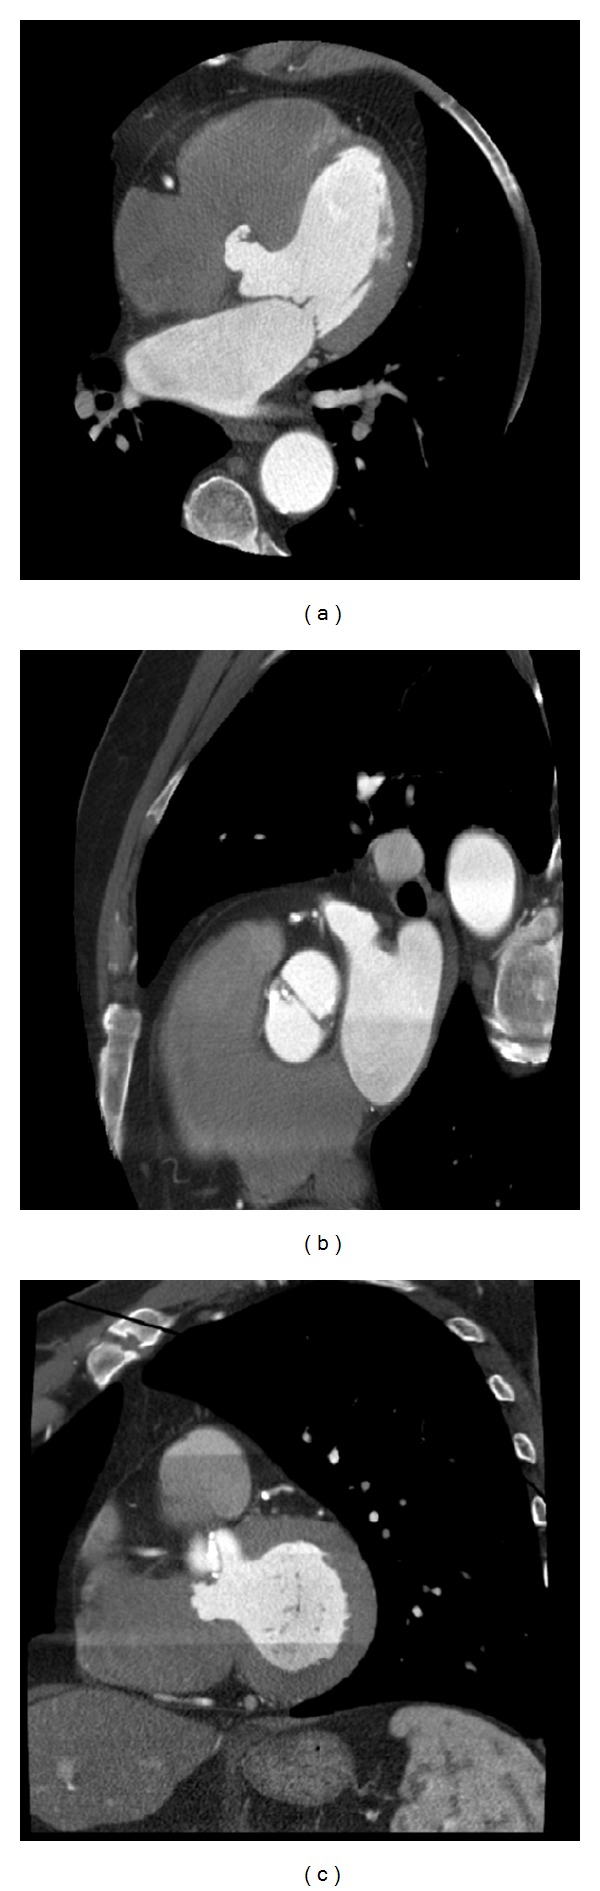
CT imaging results of the elderly patient (a) four-chamber view evidencing a membranous ventricular septal aneurysm. (b)-(c) Short axis views depicting the aneurysm.
